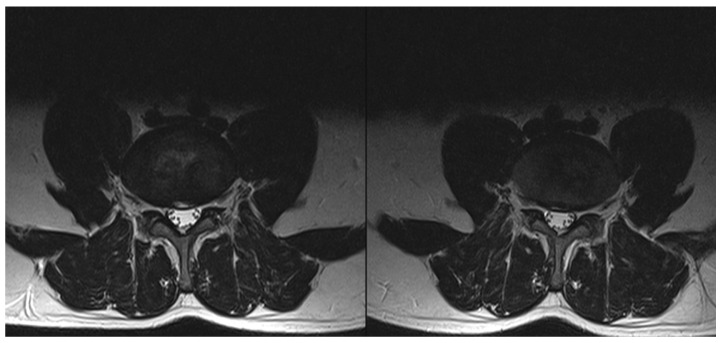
Pre- (left) and post-GEI (right) axial MRI at L4/L5 level of a patient with discogenic pain. MRI was performed two weeks before and six weeks after the GEI.
